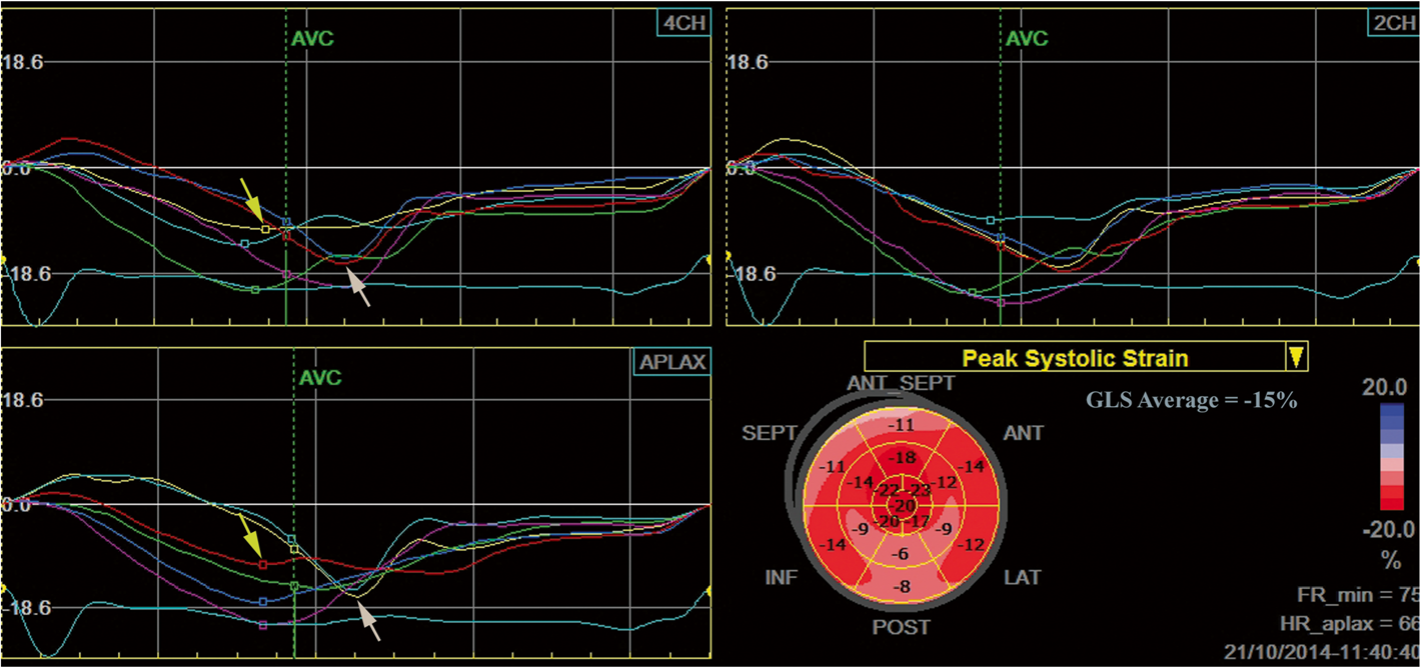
Left ventricular longitudinal strain measured by speckle tracking echocardiography in a patient with severe aortic stenosis and chest pain. A nonuniform reduction of longitudinal deformation can be observed, with reduced values of peak systolic strain in the basal segments of the interventricular septum (yellow arrows) and post-systolic shortening in mid and basal segments of the lateral wall (white arrows). Coronary angiography revealed a calcified left main stenosis (80%) extended to the origin of the circumflex artery and a hypoplastic right coronary artery.
